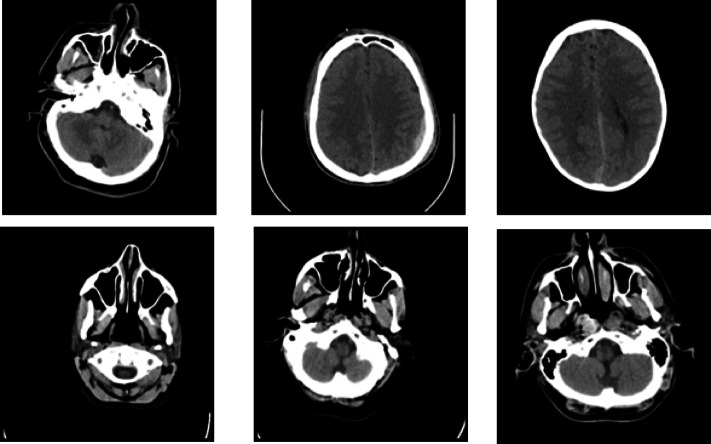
Representative CT scan slices from the dataset showing varying brain tumor locations, shapes, and intensities, providing a diverse foundation for robust feature extraction and model training.
